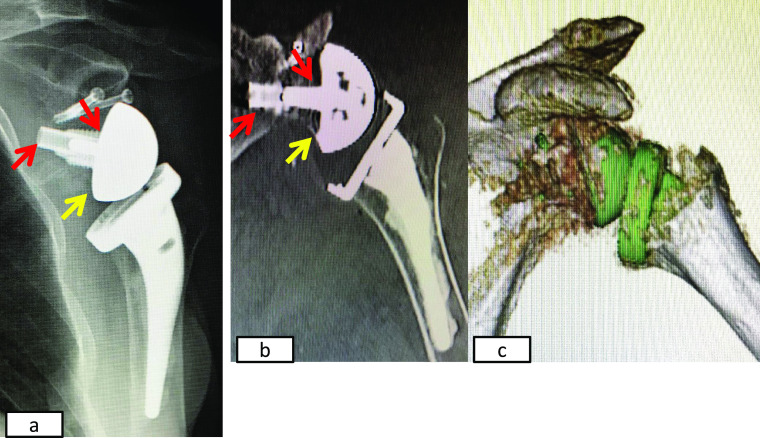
Images obtained 2 months after the final operation. a) Plain radiography. b) Coronal multiplanar CT showing the baseplate with a long
